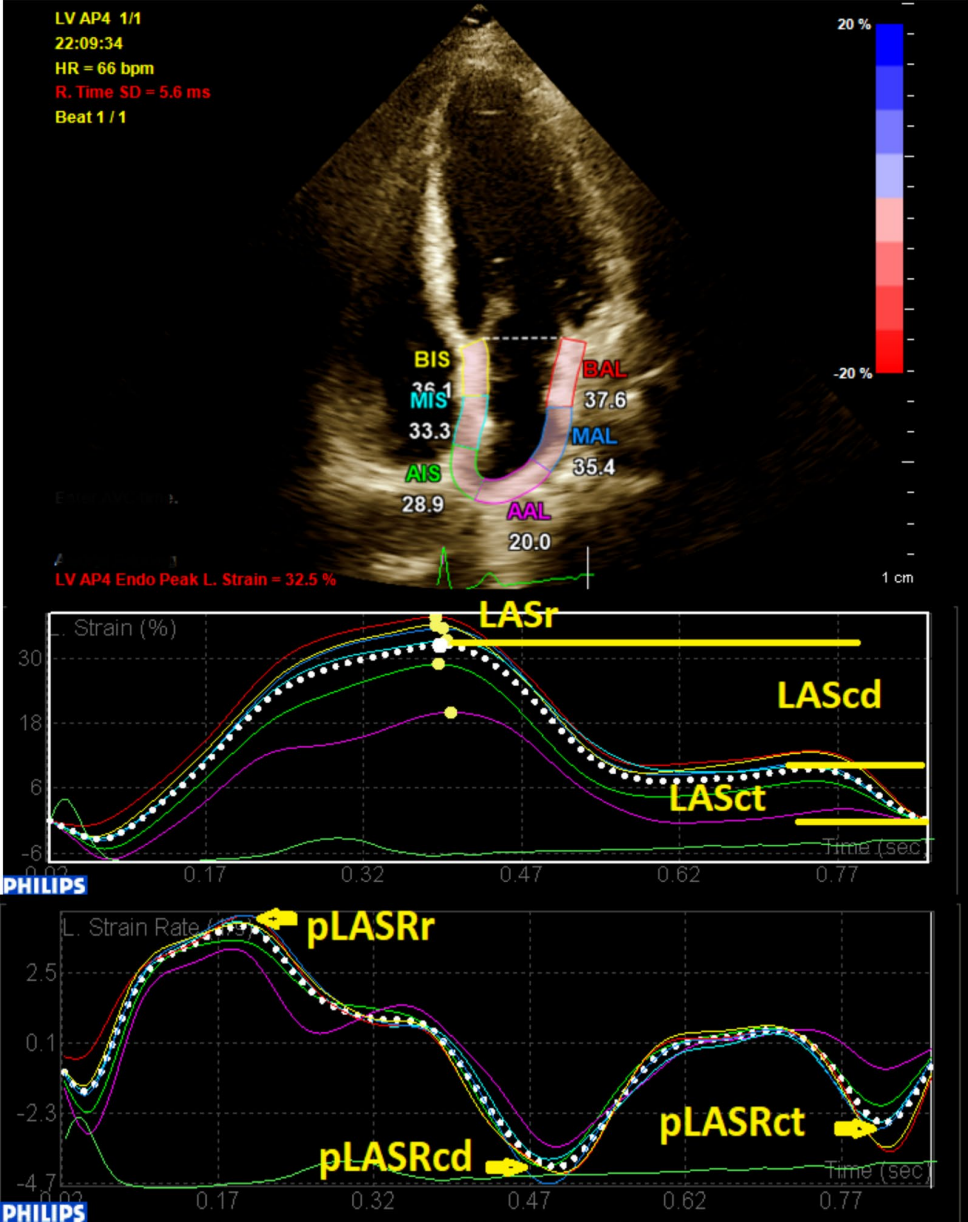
Two-dimensional speckle-tracking echocardiography for the evaluation of left atrial myocardial function is presented in the apical 4-chamber view. LAScd, Left atrial longitudinal strain during the conduit phase; LASct, Left atrial longitudinal strain during the conduit phase; LASr, Left atrial longitudinal strain during the reservoir phase; pLASRcd, Peak left atrial longitudinal strain rate during the conduit phase; pLASRct, Peak left atrial longitudinal strain rate during the contraction phase; pLASRr, Peak left atrial longitudinal strain rate during the reservoir phase
